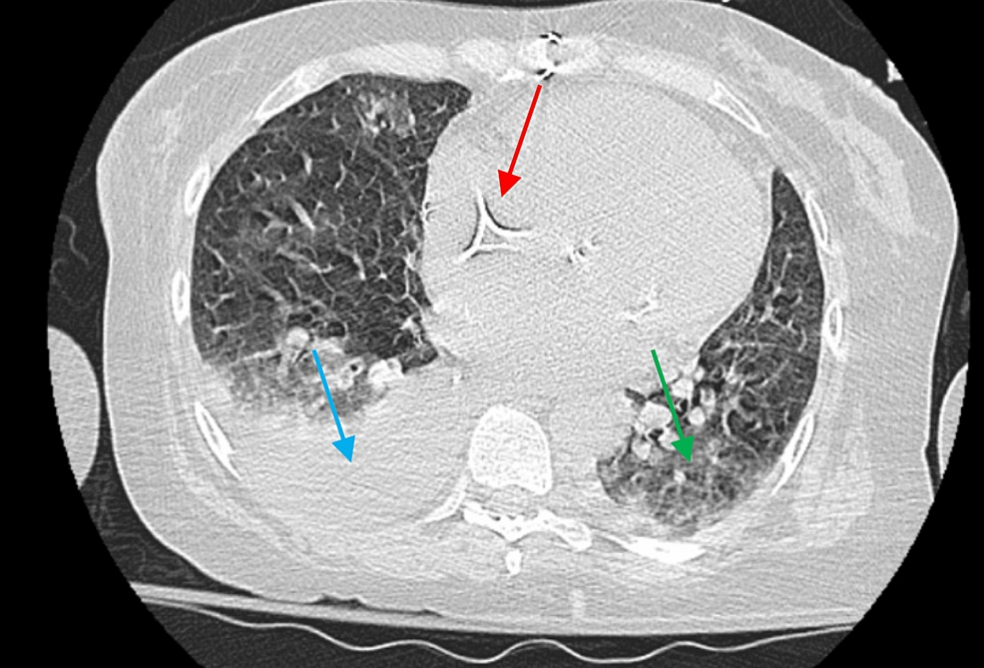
CT of the chest without contrast demonstrating right pleural effusion (blue arrow), ground-glass opacities (green arrow), and bioprosthetic mitral valve (red arrow).
